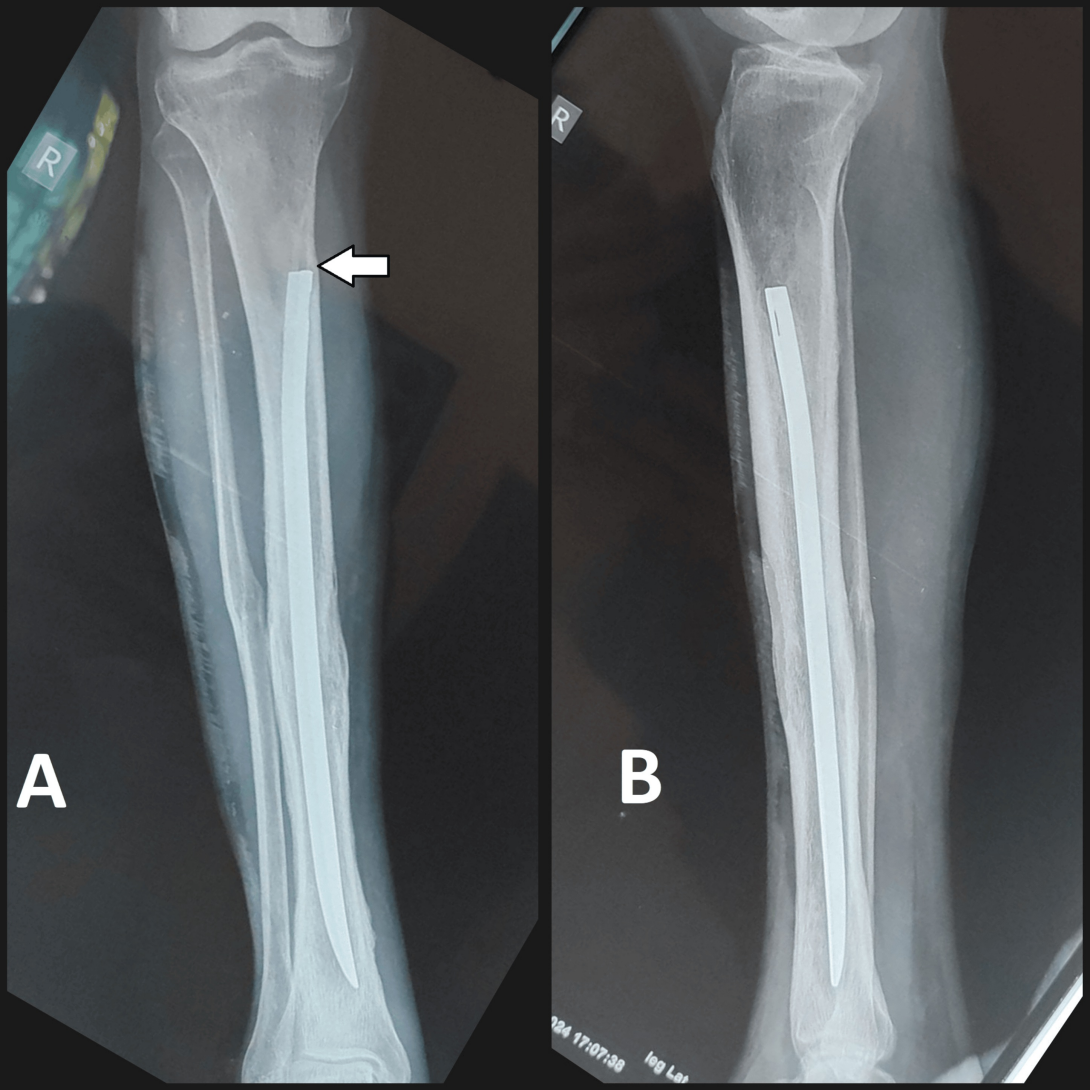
Pre-operative radiograph: (A) Antero-posterior view and (B) lateral view with V-nail in situ (white arrow showing the entry point of the V-nail)
